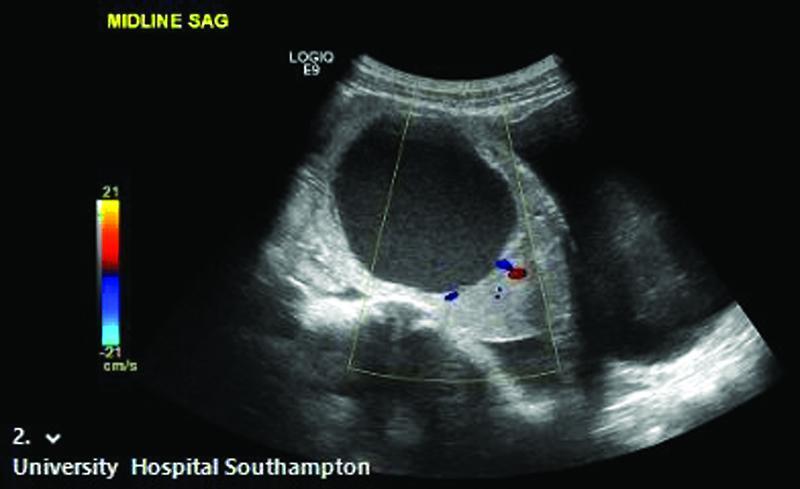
Ultrasonography demonstrating a sagittal view of suspected left adnexal mass. Laparoscopy confirmed that the tumor was in fact associated with the right ovary.
